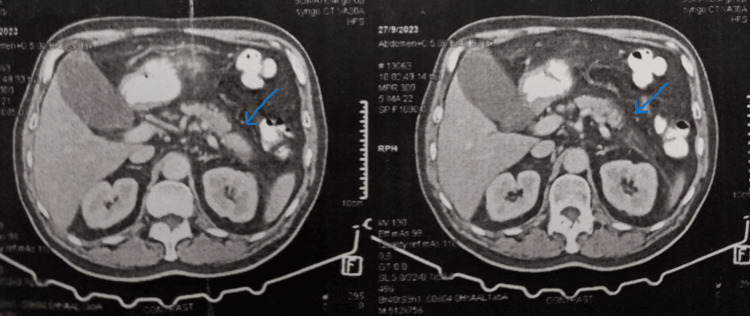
CT scan of the abdomen depicting inflammation of the pancreatic tail CT: computed tomography
